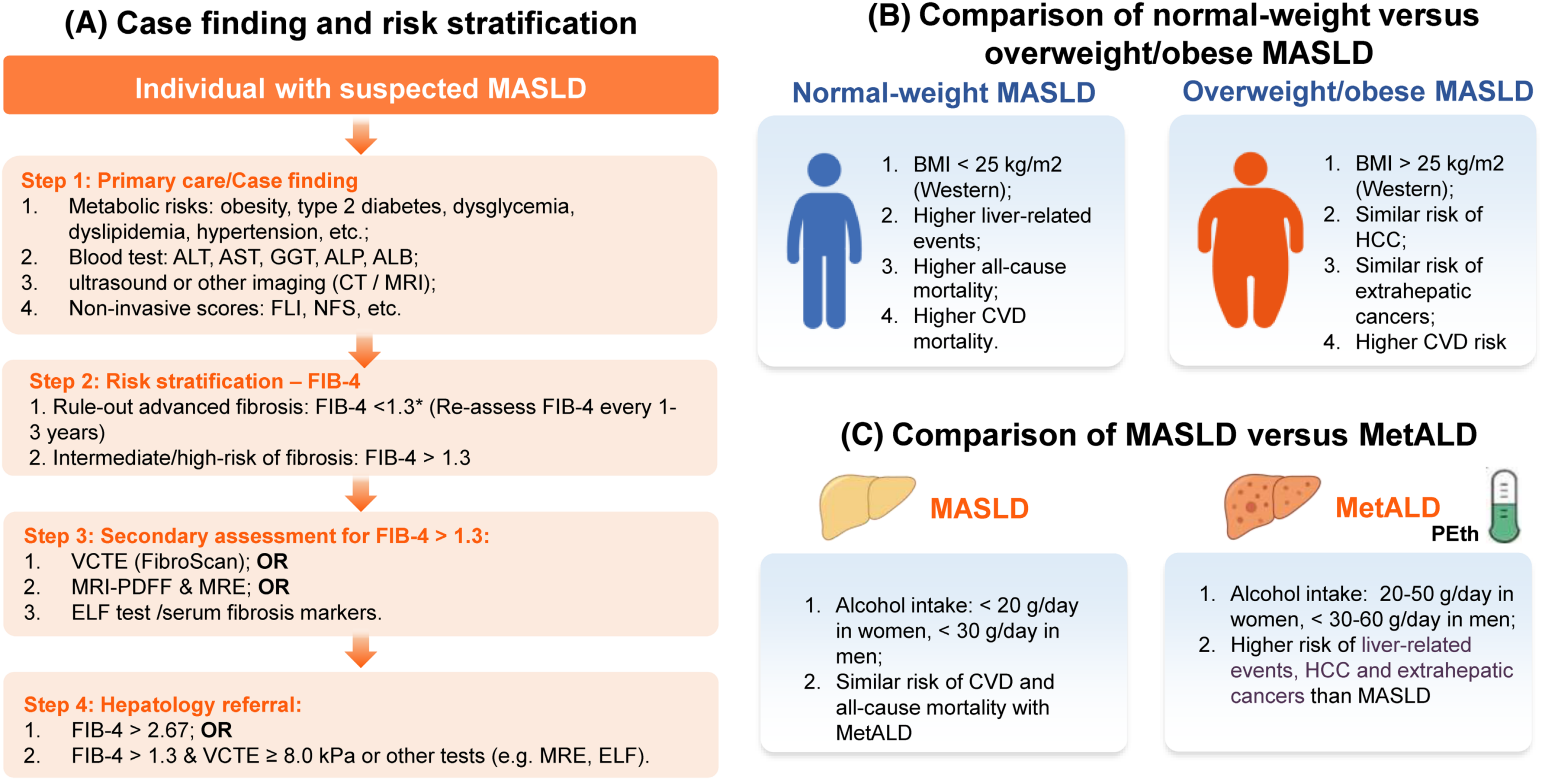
Key clinical assessment of MASLD. (A) Case finding and risk stratification: initial evaluation of individuals with metabolic risk factors includes liver enzymes, metabolic profiling, and non‐invasive fibrosis scoring (e.g., FIB‐4), followed by VCTE or serum fibrosis markers for indeterminate or high‐risk cases. This stepwise approach supports early identification of individuals at risk for advanced fibrosis. (B) Comparison of normal‐weight versus overweight/obese MASLD: normal‐weight MASLD (normal BMI) and overweight/obese MASLD present distinct metabolic and clinical characteristics. Normal‐weight MASLD is associated with higher risks of liver‐related events, CVD mortality and all‐cause mortality, whereas overweight/obese MASLD is more strongly linked to systemic metabolic dysfunction. Recognition of these phenotypic differences is critical for accurate diagnosis, metabolic evaluation, and individualised management. (C) Comparison of MASLD and MetALD: MASLD (no/low alcohol intake) and MetALD (metabolic dysfunction plus moderate alcohol consumption) share overlapping features but differ in liver‐related risk. MetALD is associated with higher rates of liver‐related events, HCC and extrahepatic cancers. Objective alcohol assessment, including structured questionnaires and biomarkers such as PEth, is essential for accurate classification and appropriate therapeutic decisions. *The FIB‐4 cut‐off is age‐adjusted for age >65 years. ALP, alkaline phosphatase; ALT, alanine aminotransferase; AST, aspartate aminotransferase; BMI, body mass index; CVD, cardiovascular disease; CT, computed tomography; ELF, Enhanced Liver Fibrosis test; FIB‐4, fibrosis‐4 index; FLI, fatty liver index; GGT, γ‐glutamyltransferase; HCC, hepatocellular carcinoma; MASLD, metabolic dysfunction–associated steatotic liver disease; MetALD, metabolic and alcohol‐associated liver disease; MRE, magnetic resonance elastography; MRI‐PDFF, magnetic resonance imaging–proton density fat fraction; NFS, NAFLD fibrosis score; PEth, phosphatidylethanol; VCTE, vibration‐controlled transient elastography.
